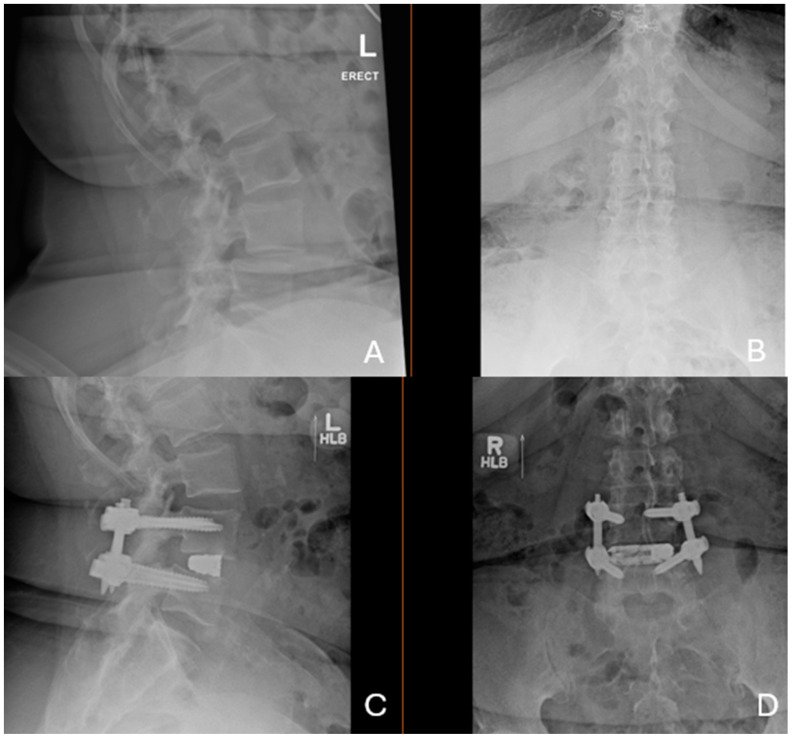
68-year-old female with a mobile spondylolisthesis and radiating leg pain. (A): Preoperative lateral radiograph demonstrating an L4–5 spondylolisthesis. (B): Preoperative AP radiography demonstrating normal coronal alignment. (C): 6-month lateral postoperative radiograph after a minimally invasive transforaminal lumbar interbody fusion with posterior instrumentation and fusion demonstrating complete spondylolisthesis reduction with disc height restoration. (D): 6-month postoperative AP radiograph.
